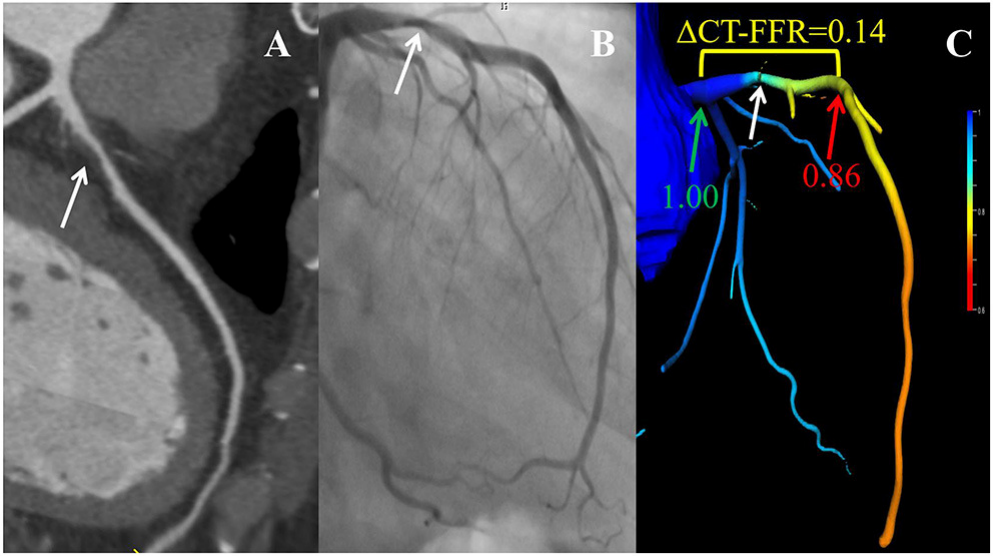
Example of a 56-year-old man with chest pain. CCTA (A) and ICA (B) as well as CT-FFR (C) showing an LAD with moderate stenosis (white arrow). ΔCT-FFR was the difference of values obtained by subtracting CT-FFR_distal_ (red arrow) from CT-FFR_proximal_ (green arrow), where CT-FFR_proximal_ and CT-FFR_distal_ were defined as the values proximal or distal within 2 cm to the lesion plaque, respectively. CCTA, coronary computed tomography angiography; ICA, invasive coronary angiography; CT-FFR, computed tomography-derived fractional flow reserve; LAD, left anterior descending coronary artery.
